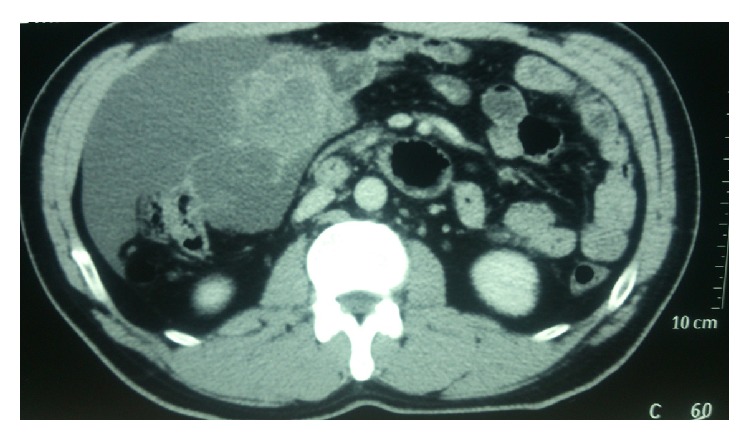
Contrast enhanced computed tomography (CECT) of abdomen and pelvis showing similar size irregular multiseptated multicystic lesion engulfing the ascending colon and caecum. Bowel lumen showed no growth. CECT abdomen showing an irregular multiseptated lesion lying over the ascending colon.
